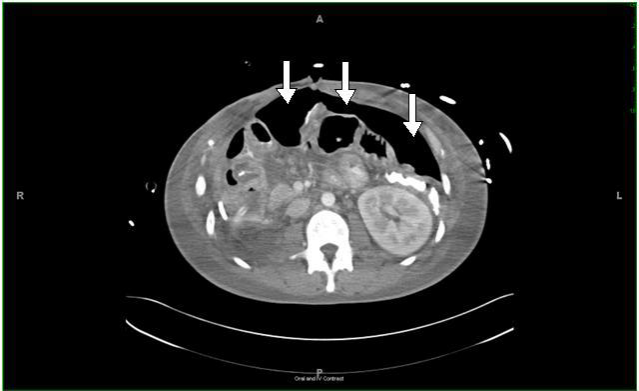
Post-operative computed tomography (CT) scan demonstrating a large volume pneumoperitoneum due to bronchoperitoneal fistula.
